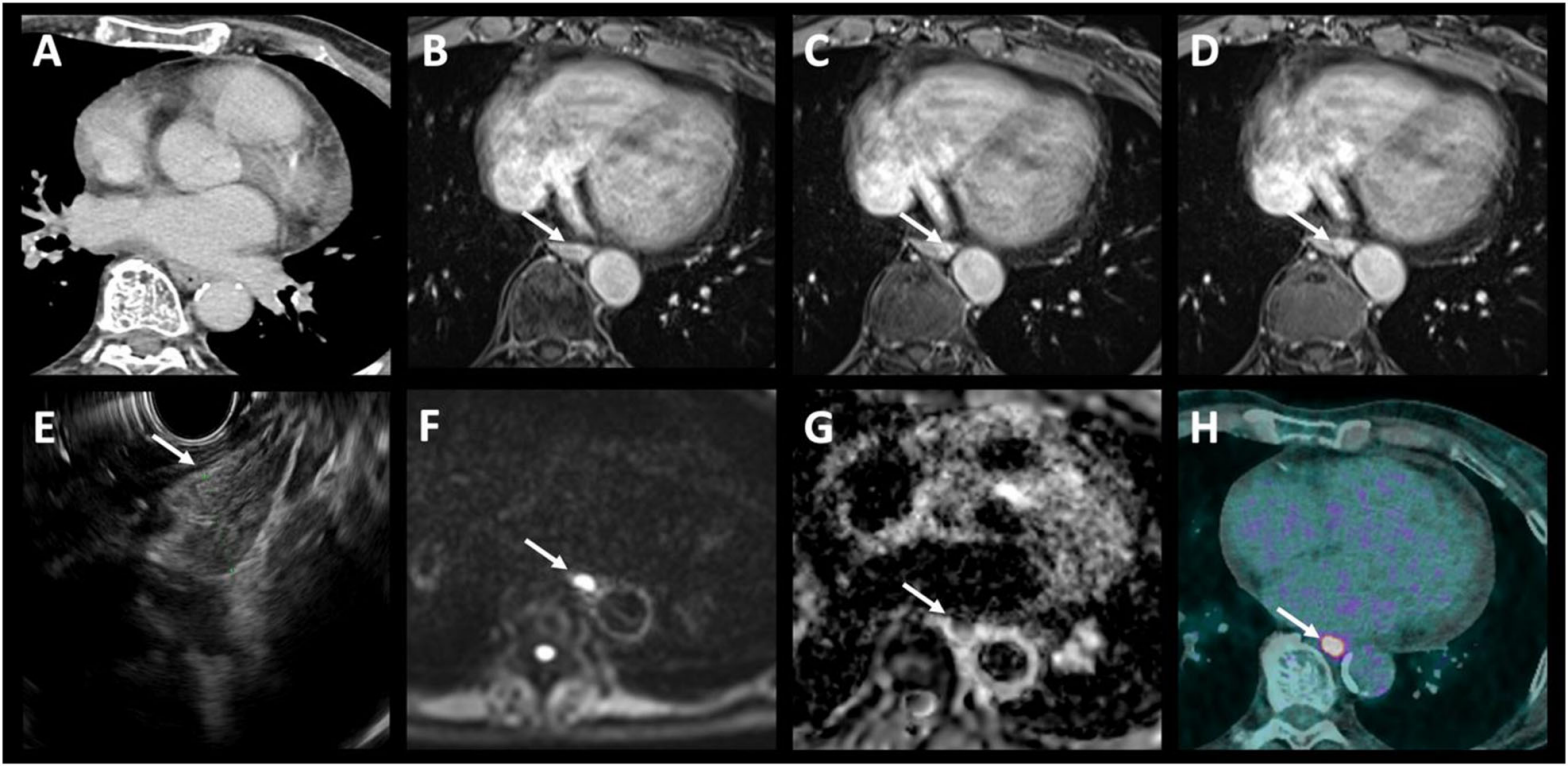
Example 2—Small tumor not detectable on CT. A 69-year-old patient with squamous cell carcinoma located in the middle third of the esophagus. The contrast-enhanced CT image (A) does not show the tumor. Contiguous enhanced-T1 MR images (B–D) show a small lesion on the posterolateral wall of the esophagus with restricted diffusion (F, G), classified T2N0. The T2 stage was confirmed by endoscopic ultrasound (E), and the absence of adenopathy and distant metastases was confirmed by PET/CT images (H)
